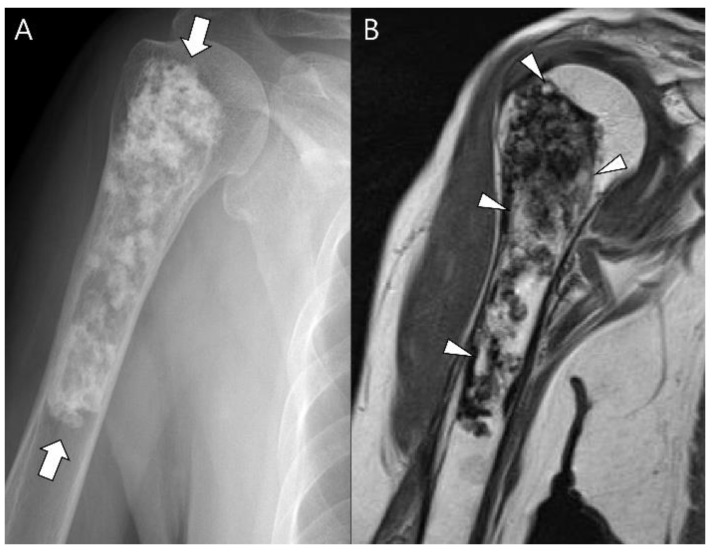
Atypical cartilaginous tumor of the humerus in an 81-year-old woman. (A) Plain radiograph demonstrates an intramedullary mass with prominent chondroid matrix mineralization (arrows) in the humerus. (B) Coronal T1-weighted image shows a lobulated intramedullary mass with areas of entrapped medullary fat (arrowheads).
